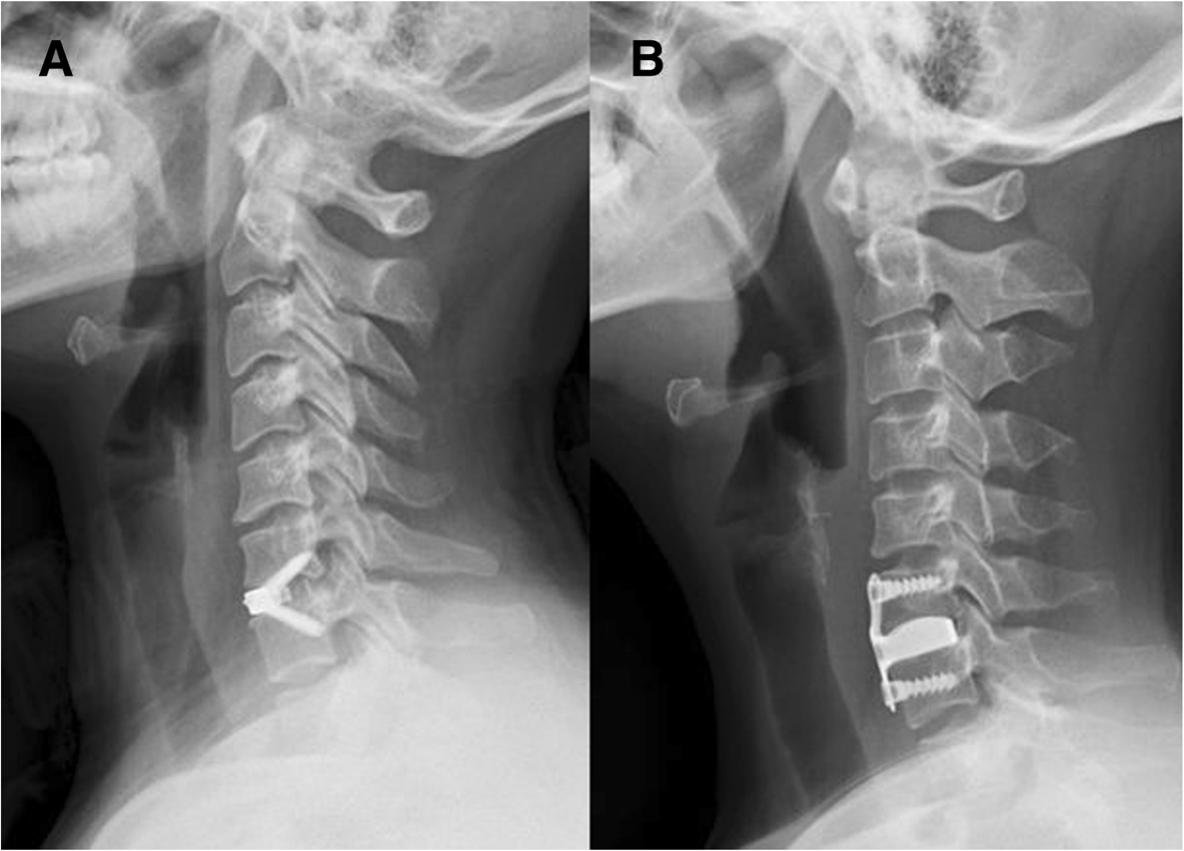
Postoperative lateral radiographs showing a a patient with C6–7 anterior cervical discectomy and fusion (ACDF) with a Zero-P implant, and b a patient with C6–7 ACDF with a PCB implant
